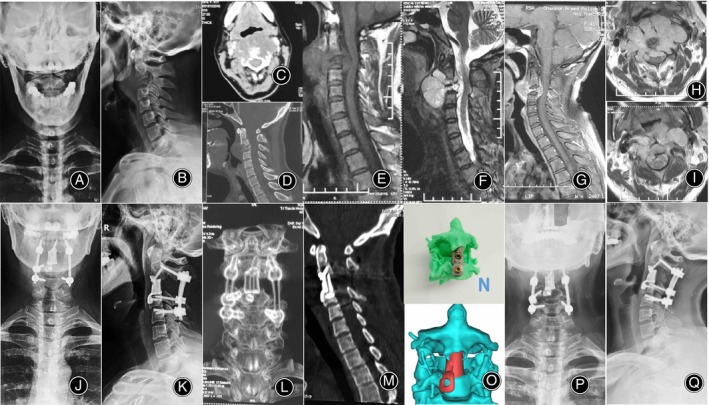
Female, 41 years old, with chordoma. (A,B) Preoperative x‐rays showing lesions in the C3 vertebra. (C,D) Preoperative CT scans revealing bone destruction in the C3 vertebra, and spinal cord compression. (E–I) Preoperative lumbar spine MRI demonstrating C3 vertebral bone destruction, formation of soft tissue tumor around, and compression of the dural sac. (J,K) The x‐ray taken 1 week postoperatively showed that the cervical pedicle screws and the 3D printed artificial vertebral body were stable. (L,M) The CT scan 1 week postoperatively showed adequate spinal canal decompression, with the 3D printed artificial vertebral body and pedicle screws remaining stable. (N,O) 3D printed model and 3D software reconstructed model. (P,Q) The x‐ray 2 years after surgery showed no significant tumor recurrence in the surgical area, and the 3D printed artificial vertebral body and pedicle screws remained stable. CT, computed tomography; MRI, magnetic resonance imaging.
